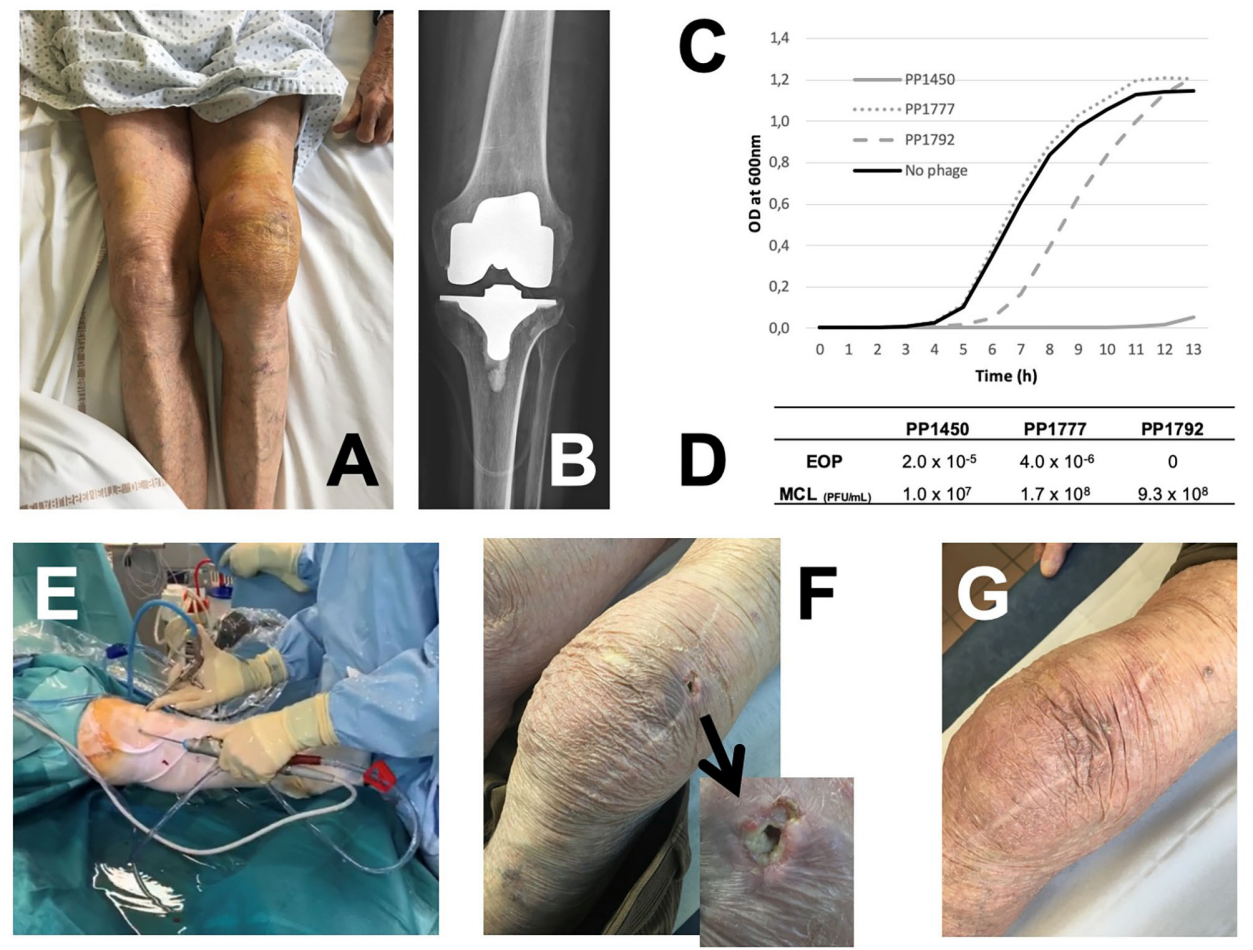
(A) Left knee joint effusion due to relapsing *P. aeruginosa* prosthesis knee infection; (B) X-ray showing no prosthesis loosening. The susceptibility of the patient's strain to the bacteriophages PP1450, PP1777, and PP1792 (phagogram) was performed using two complementary techniques: (C) For the kinetic assay, phages were incubated at a theorical multiplicity of infection (MOI, ratio of phages/bacteria) equal to 100 with the patient's strain. PP1450 was able to inhibit the bacterial growth (gray full line); PP1792 delayed the bacterial growth (gray dotted line) and PP1777 had no impact (gray dashed line). (D) For the plaque assay, titers obtained with the patient's strain and the reference strain are determined to calculate the efficiency of plating score (EOP) score (the closer to 1 is the score, the more efficient the phage is). Phages PP1450 and PP1777 were active on the patient's strain with an EOP score of 2.0 × 10^−5^ and 4.0 × 10^−6^, respectively. Partial lysis without PFU were observed for PP1792 (considered to have a weak bactericidal or bacteriostatic activity in this assay). (E) Arthroscopic DAIR with administration of the phage cocktail at the end of the procedure through the arthroscope. (F) Ulceration of a subcutaneous nodule on the external side of the knee observed 2 months after the arthroscopy. (G) Finally, a favorable outcome under suppressive antimicrobial therapy.
